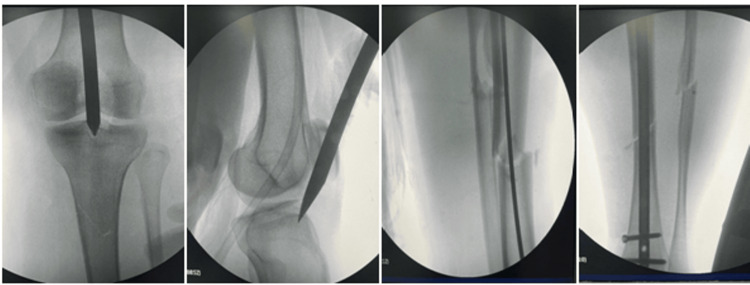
Intraoperative radiographs showing clear entry points and the introduced olive guide wire and nail in the semi-extended knee position. This patient had a proximal fracture line at the proximal tibia because of injury.
